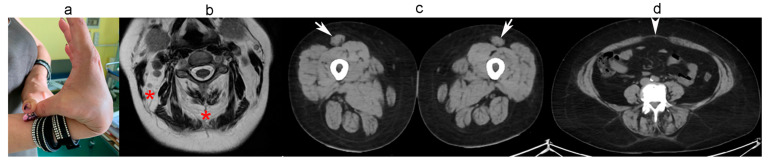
Clinical photo and muscle imaging of the 41-year-old patient from family B: (a) joint hypermobility; (b) MRI showing fibro adipose tissue between the markedly atrophic neck muscles (asterisks); (c) CT showing round bilateral hypotrophy of rectus femoris muscle (arrows). Left thigh muscles are more involved; (d) diastasis of the abdominal muscles (arrowhead), partial fibro-adipose replacement of abdominal and lumbar paravertebral muscles.
